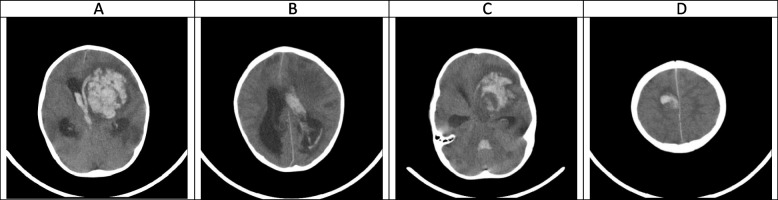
(the brain CT SCAN without contrast, the first imaging study [day 0]). A A large heterogeneous hyperdense area seen within the left frontotemporal lobes and associated with marked surrounding edema formation, suggestive of acute hemorrhage and causing significant pressure effect on the left lateral and anterior aspect of third ventricle, resulting marked shift of midline structures to the right side. Also note significant hyperdense material within lateral and third ventricles, suggestive of intraventricular hemorrhage B Sign of dilatation of lateral ventricles is seen, suggestive of associated hydrocephalus formation which is secondary to intraventricular hemorrhage and resulting communicating type of hydrocephalus. C Signs of two faint rounded hypodense lesions are seen within both cerebellar hemispheres along with other ones at the different parts of both cerebral hemispheres (not shown here) suggestive of early abscess formation. Sign of acute hemorrhage within fourth ventricle is also seen. D Sign of another smaller hyperdense lesion at the high convexity of the right parietal lobe also is seen, again suggestive of acute hemorrhage
